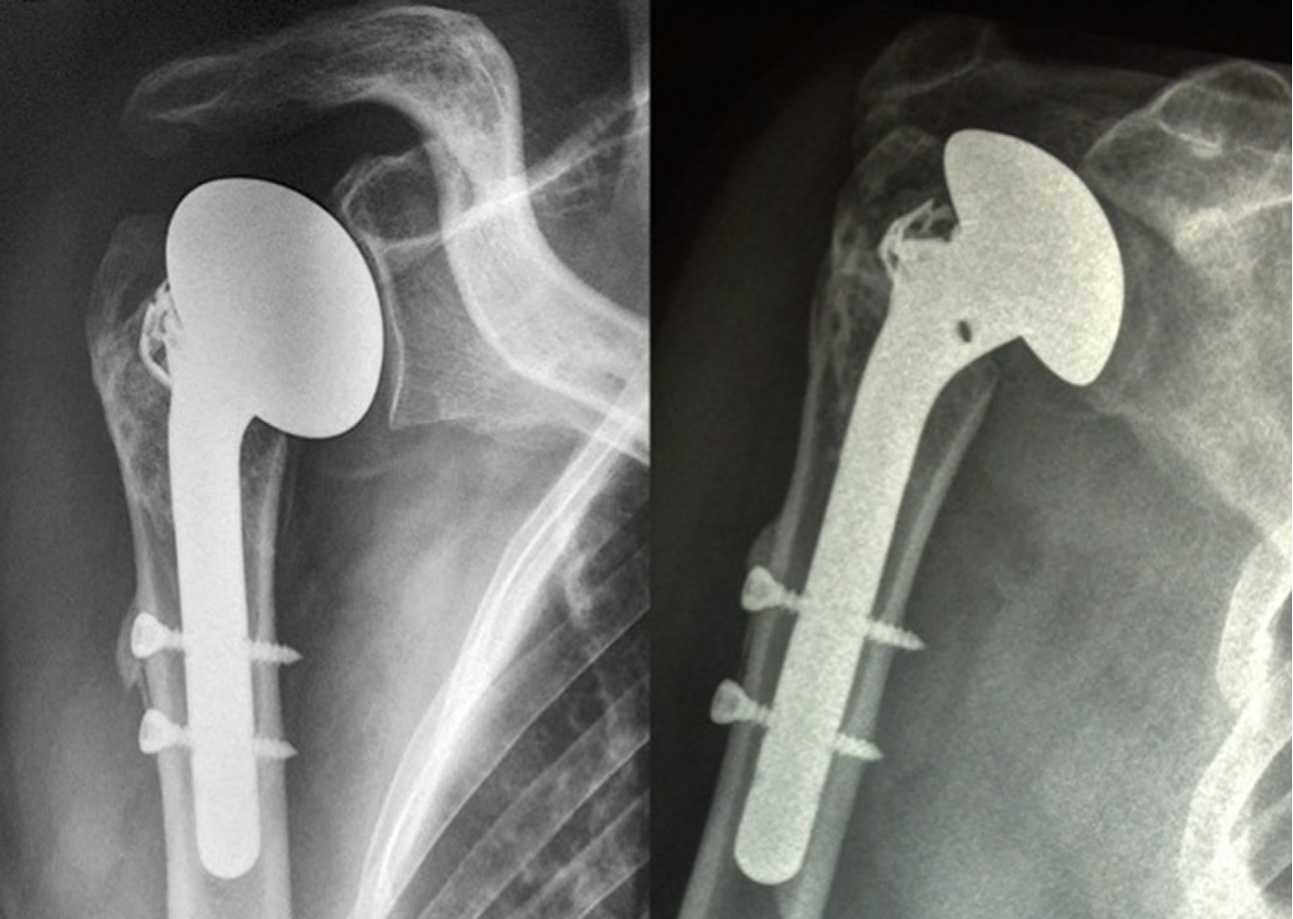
Bone union on X-ray at same follow-up.
